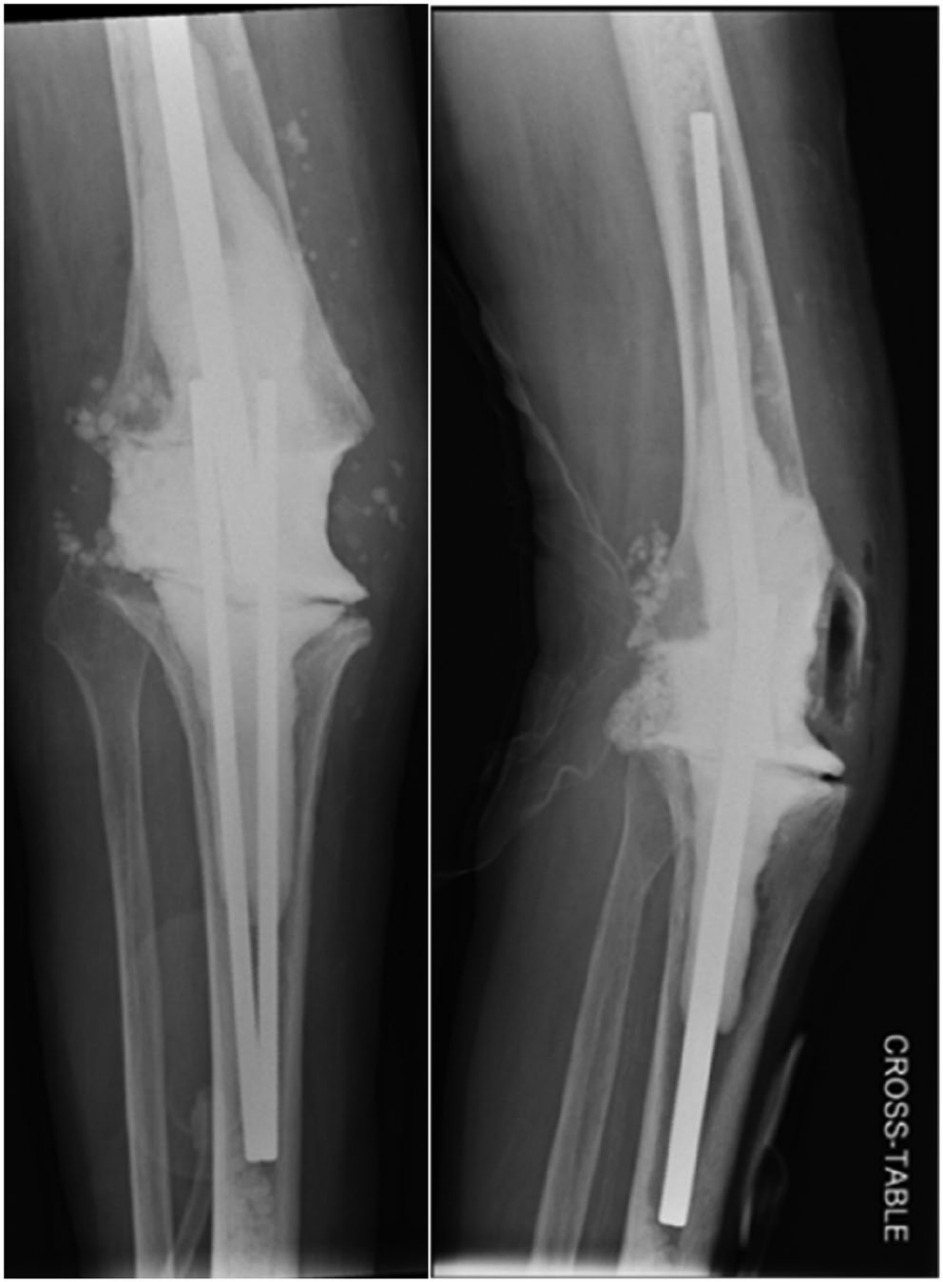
Radiograph in two planes of a static spacer constructed from Ilizarov rods
